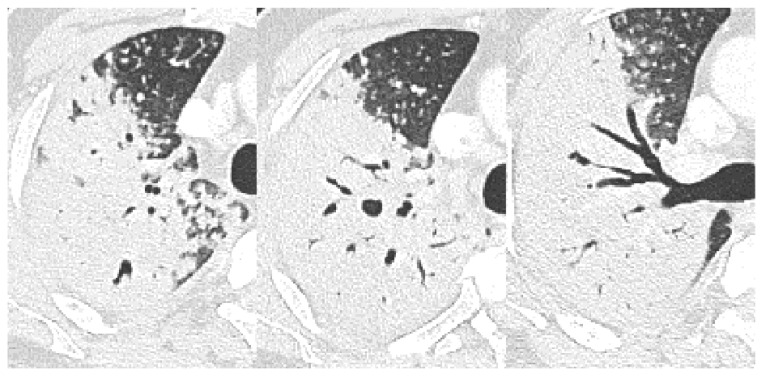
A 64-year-old male with pulmonary tuberculosis and uncontrolled diabetes (HbA1C, 7.2%). Lobar consolidation and surrounding micronodules in the right upper lobe were the main CT findings in this patient. These CT findings could be misdiagnosed as pneumonia, but cylindrical bronchial dilatations with erosive and aneurysmal changes within the consolidation helped to diagnose pulmonary tuberculosis.
